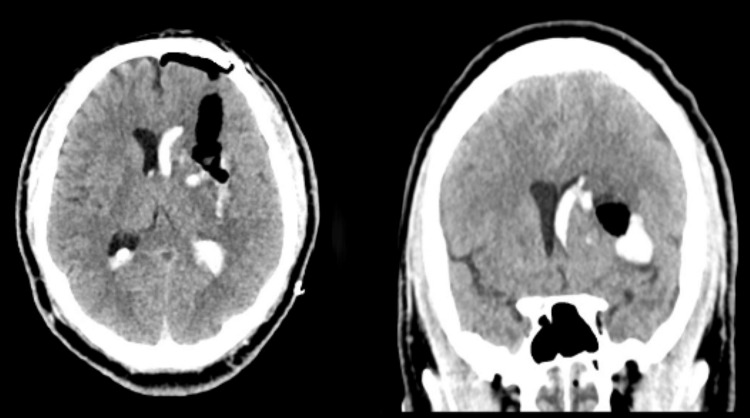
Post-operative CT scan for patient 1 CT scan with a left basal ganglia intracerebral hemorrhage (ICH)
